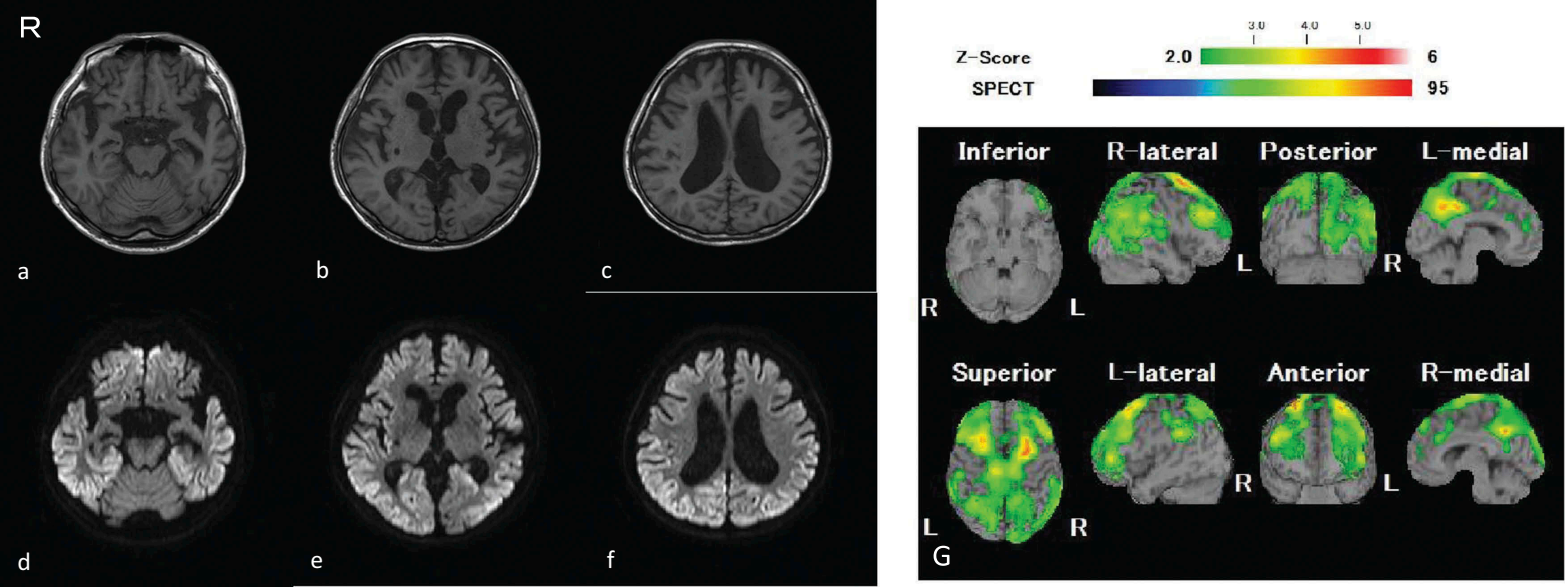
MR images and SPECT obtained 15 months after the onset of symptom. T1-weghted MR images (a-c); diffusion-weighted images (d-f), an easy Z-score (eZIS) analysis of ^9m^Tc-etyhlcysteinate dimer-single photon emission computed tomography (^99m^Tc-ECD-SPECT) image (g). The Z-score scale of 2.0 to 6 is indicated by a green to red (lower regional cerebral blood flow (rCBF)) color gradient in panel g. R: right side; L: left side. T1-weighted MRI showed bilateral frontotemporal atrophy and symmetric enlarged lateral ventricles predominantly posterior horn due to occipital atrophy (a-c). Diffusion-weighted MRI showed cortical hyper-intensities in the bilateral frontal, temporal parietal and occipital cortices without obvious signal changes in the basal ganglia 15 months after the initial symptom (d-f). An eZIS analysis of ^99m^Tc-ECD-SPECT showed decreased rCBF in the bilateral frontal, parietal, and right posterior cortices 15 months after the onset (g).
